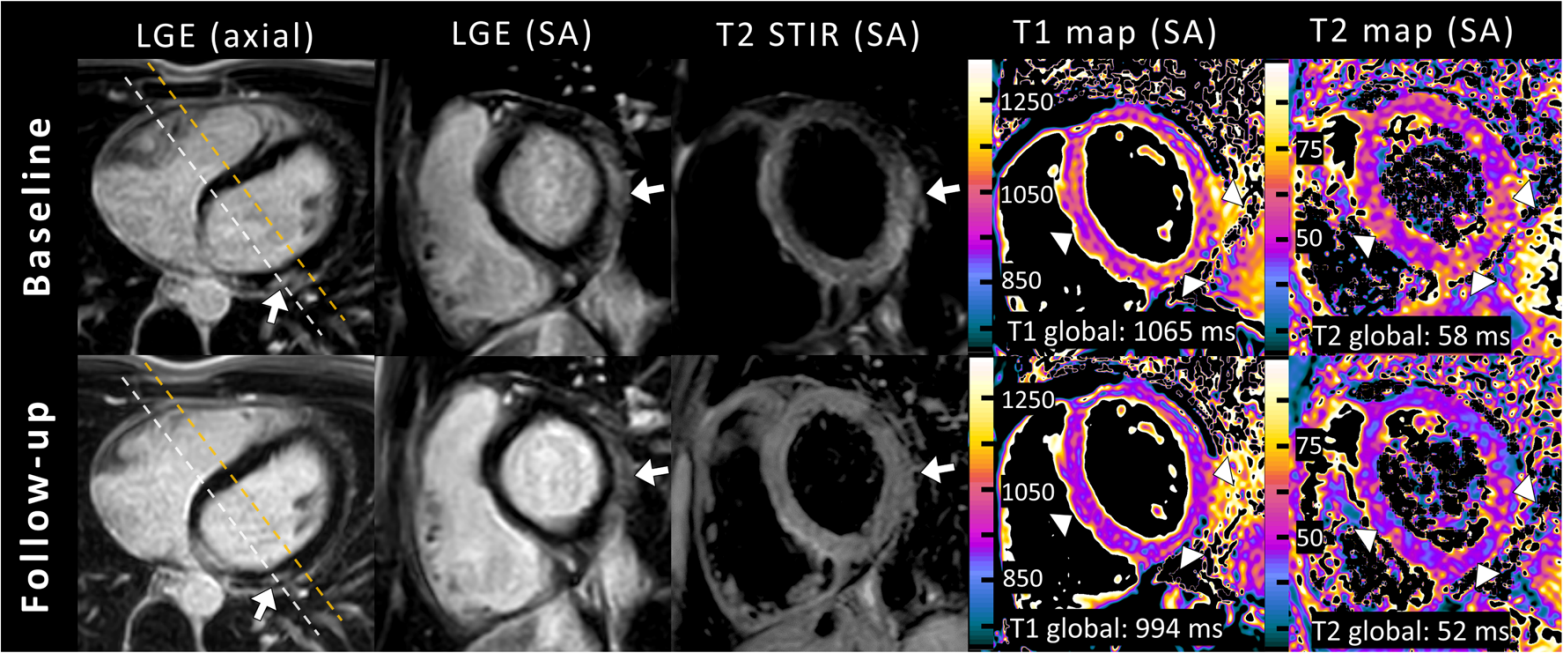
A clinical example of cardiac magnetic resonance imaging (MRI) in a 15-year-old boy with the typical appearance of acute myocarditis on cardiac MRI at baseline and with recovery on follow-up after 2 months. Cardiac MRI in end-diastole shows subepicardial enhancement of the basal lateral wall on late gadolinium enhancement (LGE) images in axial and short-axis (SA) orientation with associated focal myocardial edema (*arrows*) on fat-suppressed (T2-weighted short TI inversion recovery [T2 STIR]) images (*white dashed lines* in axial LGE images represent the imaging plane of LGE and T2 STIR). T2 STIR images at follow-up show normalization of focal myocardial edema. Mapping parameters displayed high myocardial native T1 and T2 relaxation times at baseline cardiac MRI and normalization at follow-up. Notably, in this example, T1 and T2 maps cover the transition between the basal and mid segments outside the visible late gadolinium enhancement lesions (*orange dashed lines* in axial LGE images represent the imaging plane of the quantitative maps) and detected additionally diffuse myocardial alterations in the septal and inferior wall, which normalized at follow-up (*arrowheads*)
